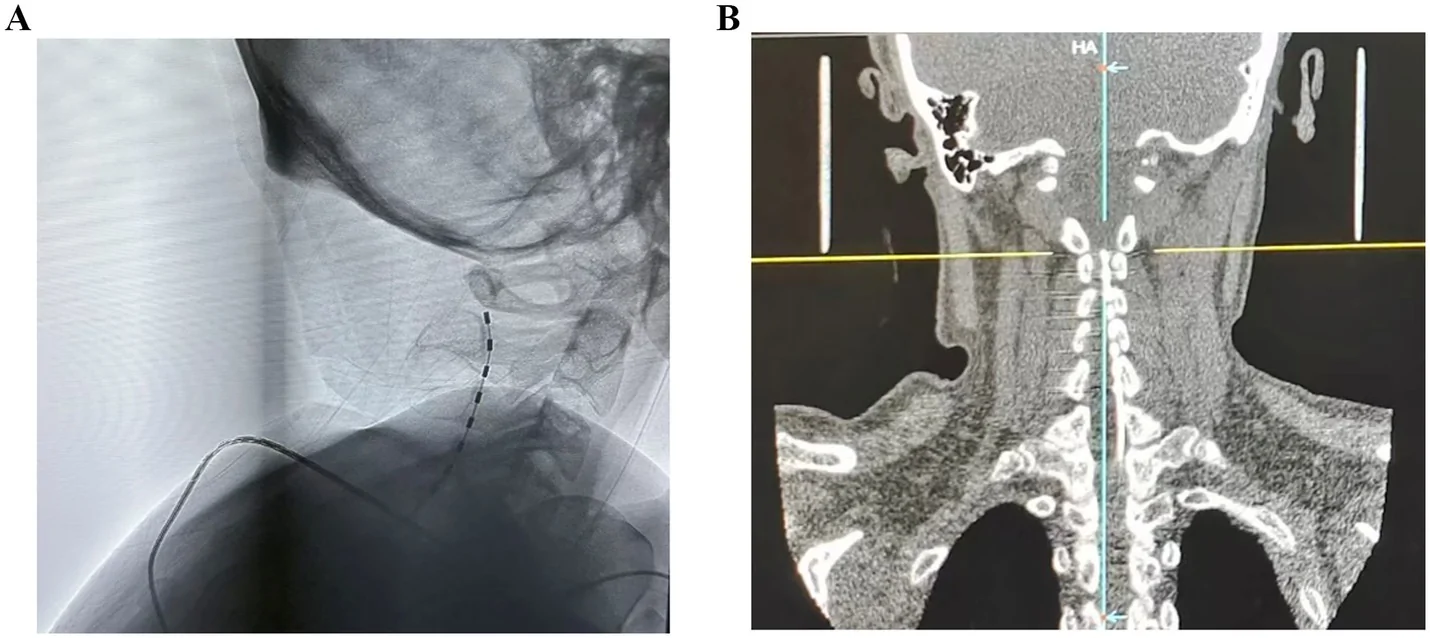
(A) Intraoperative lateral digital radiography image. (B) Postoperative coronal t CT image of the cervical spine (tissue window).
